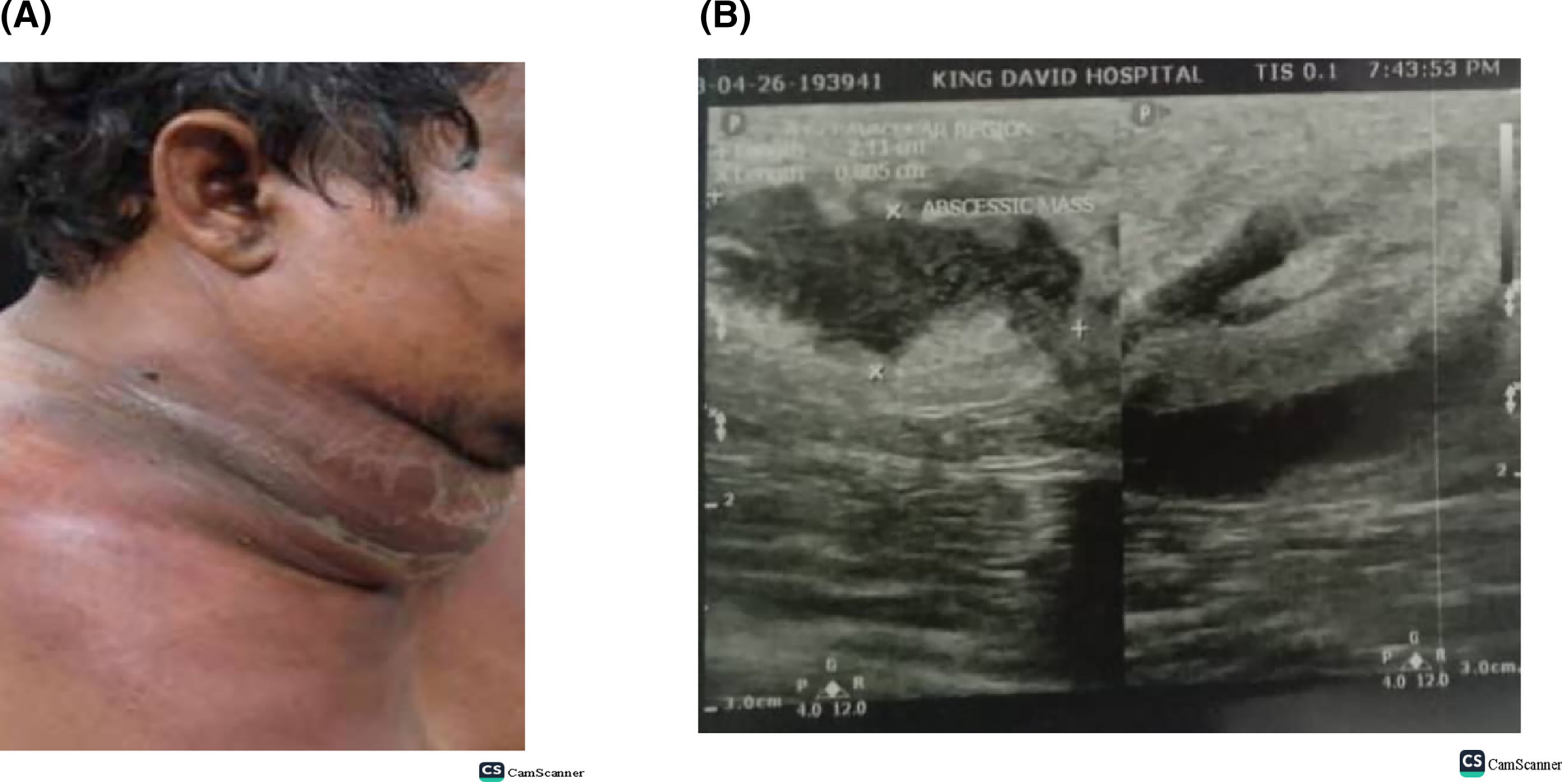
(A) visible anterior neck swelling. (B) Ultrasound scan shows extensive submandibular abscisic mass. 10.0 cm × 3.0 cm in widest dimensions. Largest pockets measuring 2.11 × 0.8 cm. Skin hyperpigmentation.
